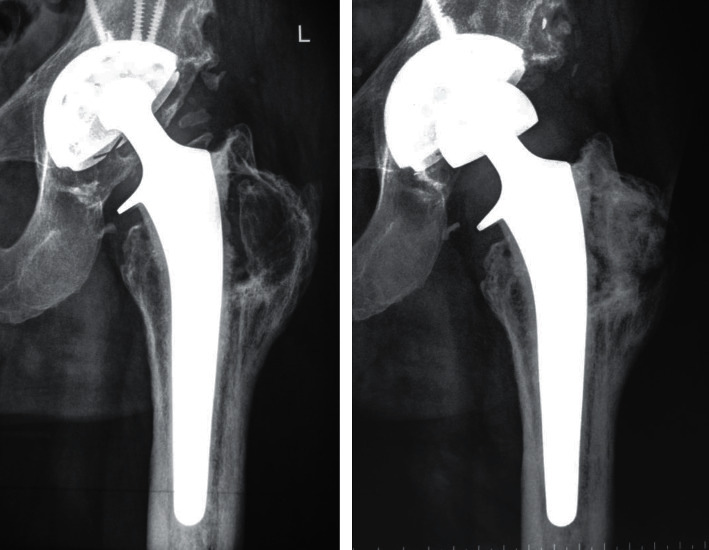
X-ray prior to the second revision showing major osteolysis of the greater trochanter due to PE insert wear (a) (2009); situation after the second revision surgery, in which the greater trochanter was bone grafted, screws were removed, and a 36 mm PE liner was cemented into the uncemented cup (b) (2012).
